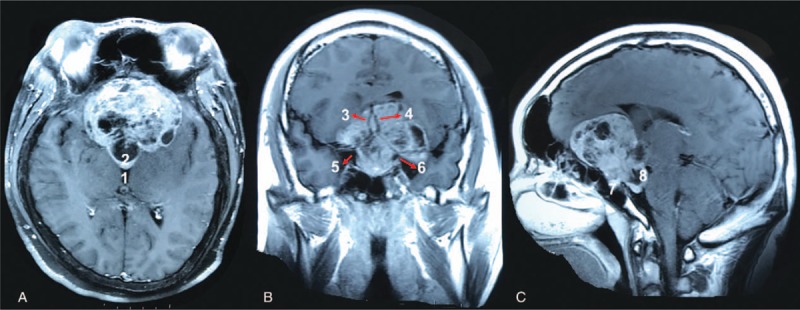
Preoperative magnetic resonance image (MRI) of the pituitary adenomas. The contrast image was presented in axis (A), coronal (B), and sagittal (C) view. 1, third ventricle. 2, The tumors invaded into the third ventricle. 3, A2 segment of the right anterior cerebral artery (ACA). 4, A2 segment of the left ACA. 5, Bifurcation of right internal carotid artery (ICA). 6, Bifurcation of left ICA. 7, Intrasellar tumors. 8, Tumors located at the interpeduncular fossa.
